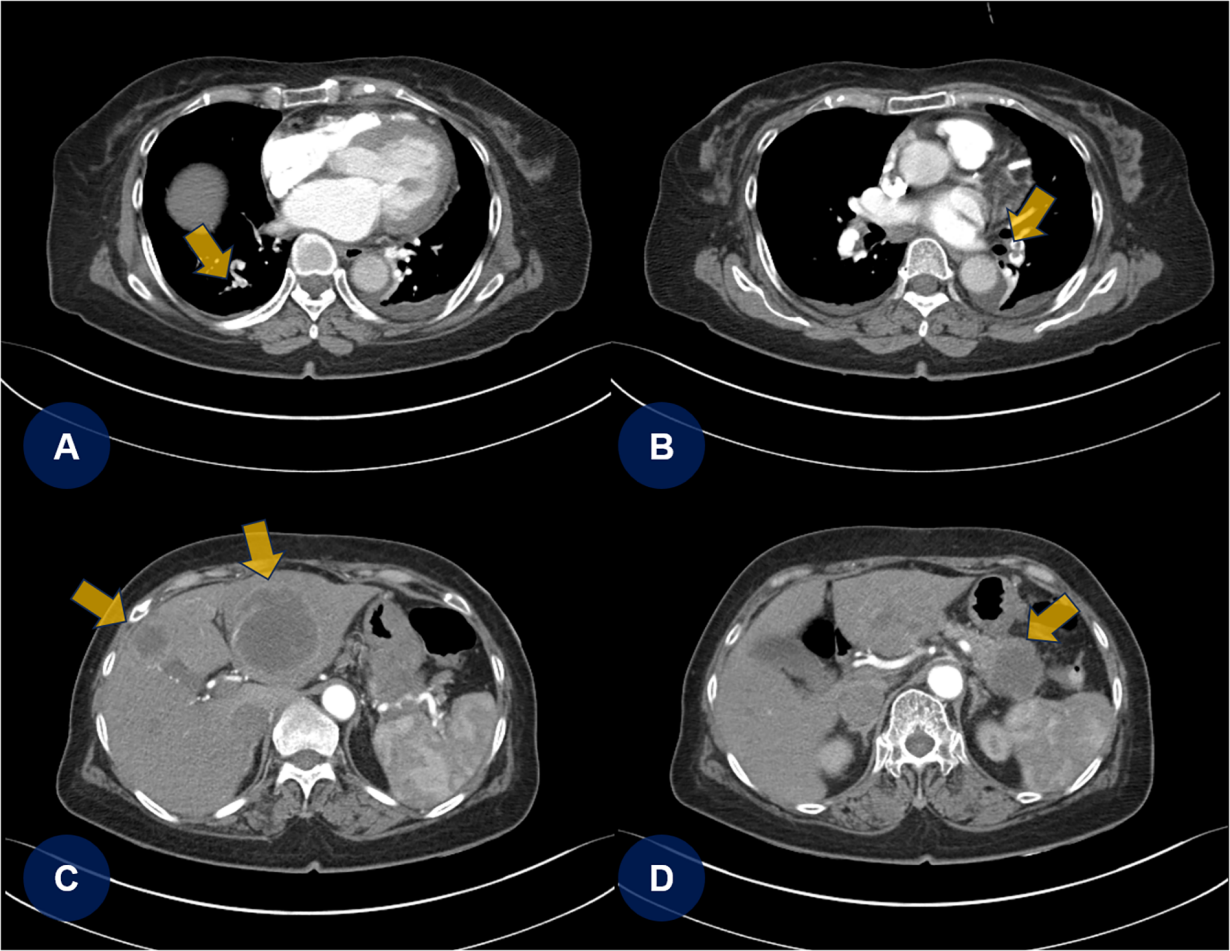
Chest and abdominal CTA. (A,B) Chest CTA reveals multifocal pulmonary thromboembolisms (yellow arrows). (C,D) Abdominal CTA shows multifocal round-shaped hepatic lesions with a solitary mass-like lesion in the tail of the pancreas. CTA, computed tomography angiography; PTE, pulmonary thromboembolism.
